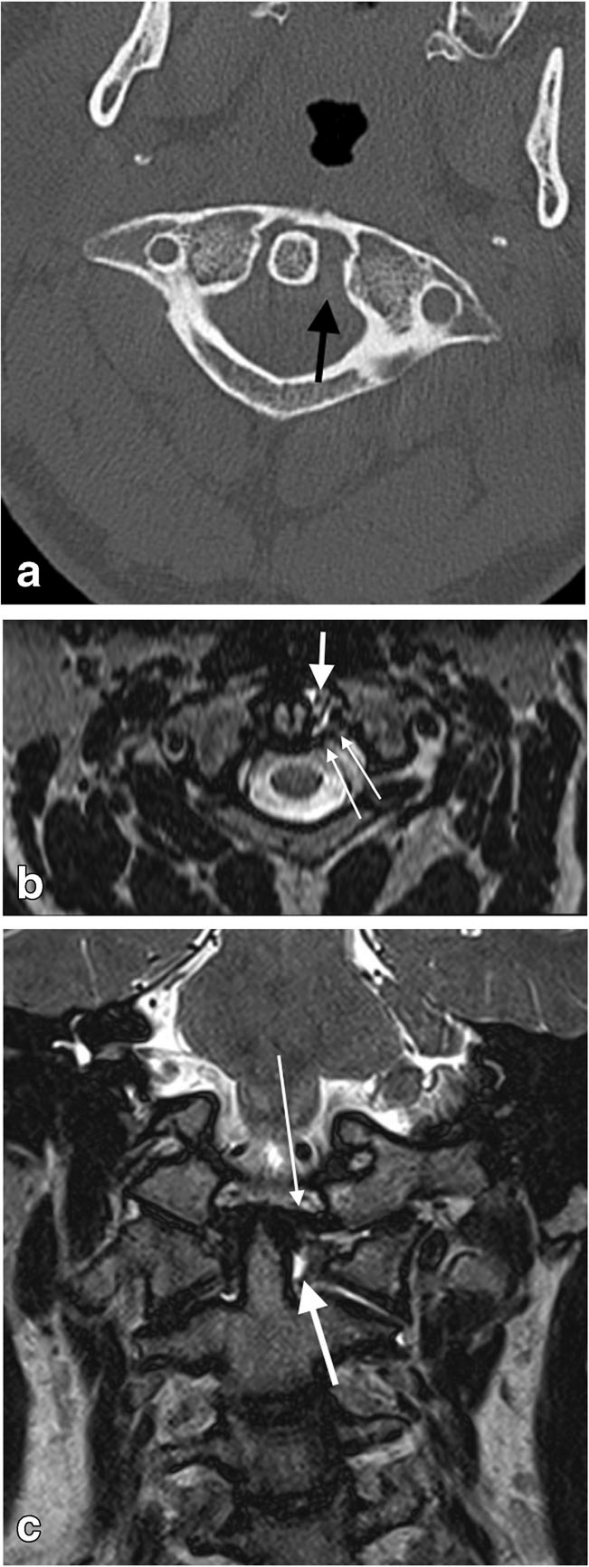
Craniocervical ligament injury in the absence of osseous injury in a 28-year-old male who sustained unimpeded fall from 3 metres associated with head injury and neck pain. There was no fracture evident on CT assessment of the craniocervical junction and sub-axial cervical spine: a axial CT image through the C1-C2 level of the craniocervical junction—there is widening of the left lateral atlanto-dental interval (*black arrow*) despite the absence of any fractures, raising the likelihood of craniocervical ligamentous injury; b reconstructed axial T2-SPACE MR image through the C1-C2 level of the same patient demonstrates disruption of the left transverse (atlantal) ligament (*thin long white arrows*) and localised haemorrhagic effusion in the widened left lateral atlanto-dental space; c coronal T2-SPACE MR image through the craniocervical junction of the same patient—the abnormal signal change related to the disrupted left transverse (atlantal) ligament and surrounding effusion is demonstrated (*thick white arrow*). The left alar ligament is mildly but abnormally stretched (*thin white arrow*). Small atlanto-axial and atlanto-occipital joint effusions are present (not annotated)
